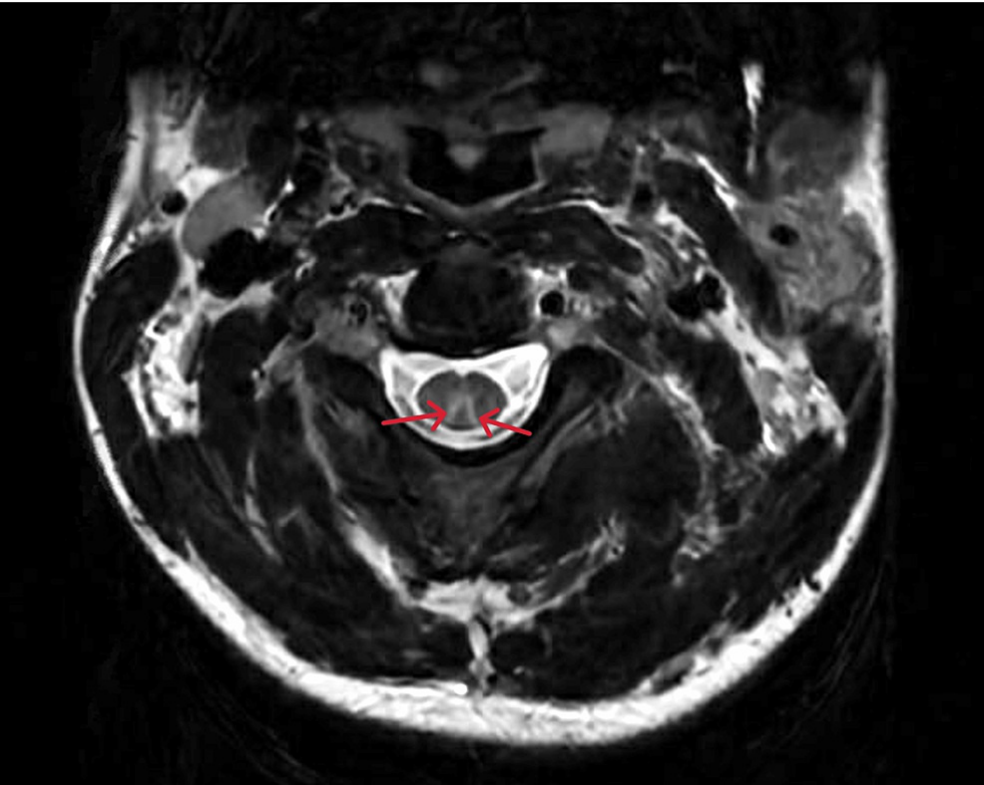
Axial MRI of the cervical spine demonstrating a T2 symmetric bilateral high intensity in the dorsal columns in the form of an inverted ‘V’ Red arrows point to the high-intensity regions.
